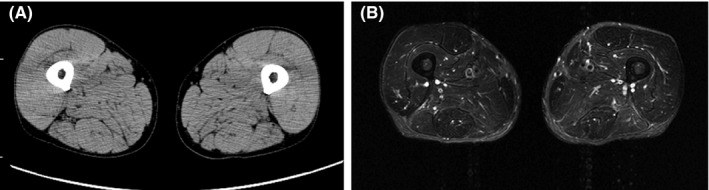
Leg muscle imaging. Computerized tomography (CT) (A), T2‐weighted short‐tau inversion recovery magnetic resonance imaging (MRI) (B). CT and MRI scans did not show any abnormalities
